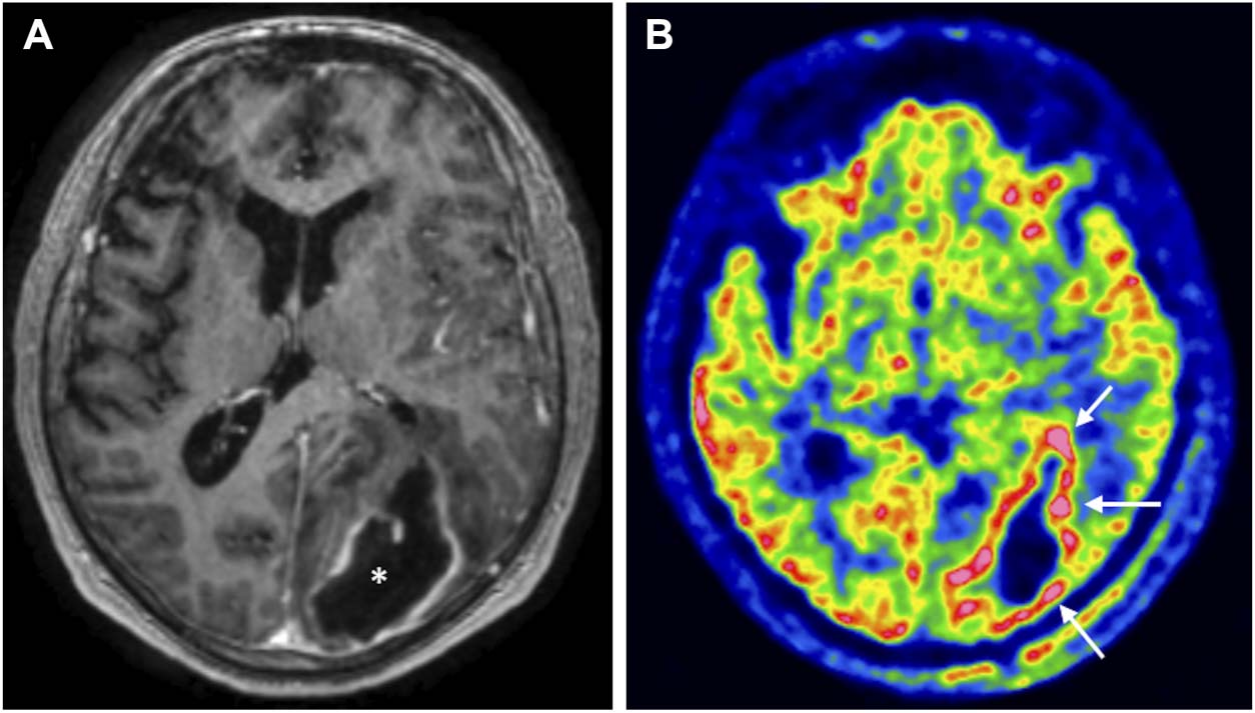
FDG uptake in the abscess capsule. A, A patient with a large abscess in the left occipital lobe (asterisk; T1-weighted MRI). B, [^18^F]-fluoro-2-deoxy-D-glucose positron emission tomography/computed tomography obtained 2 days after pus removal. There is FDG uptake corresponding to the brain abscess capsule (arrows). This finding implies the presence of leukocytes in the capsule that convert FDG to FDG-6-phosphate. FDG, [^18^F]-fluoro-2-deoxy-D-glucose.
